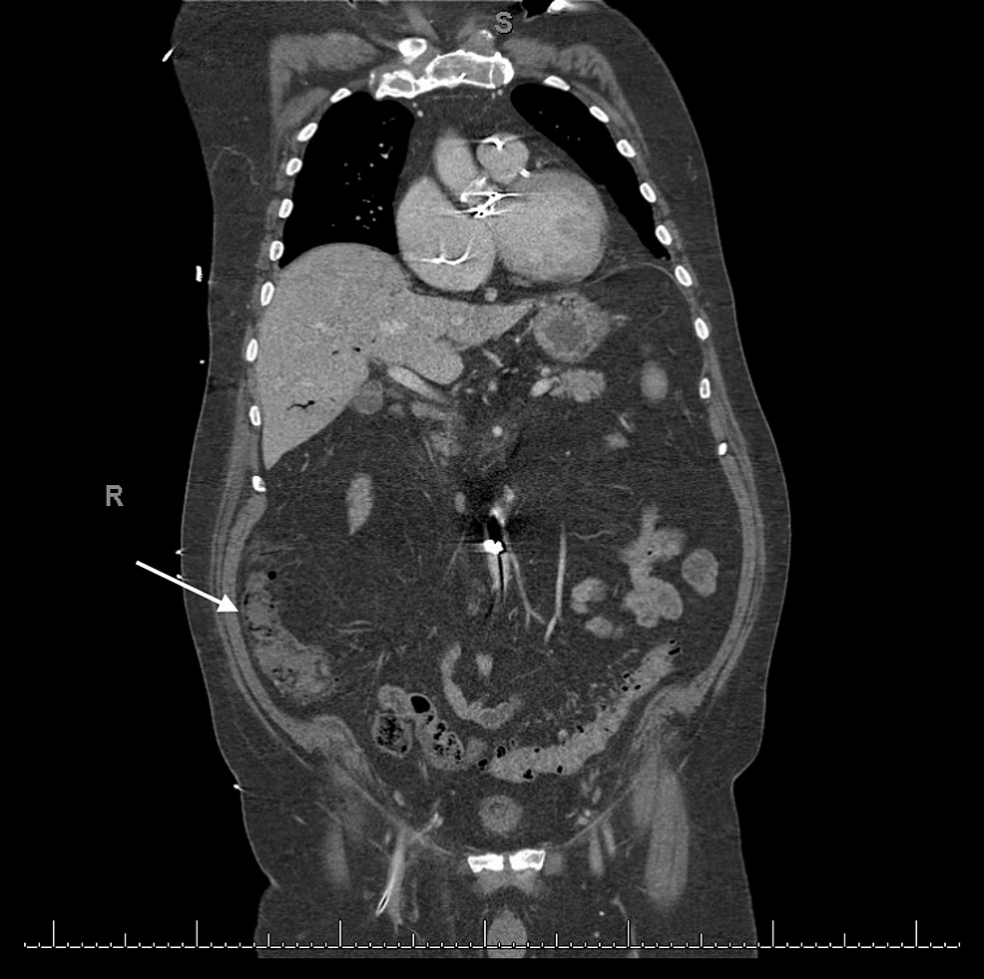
Computed tomography of chest and abdomen Computed tomography of chest and abdomen with air around the ascending colon (arrow)
